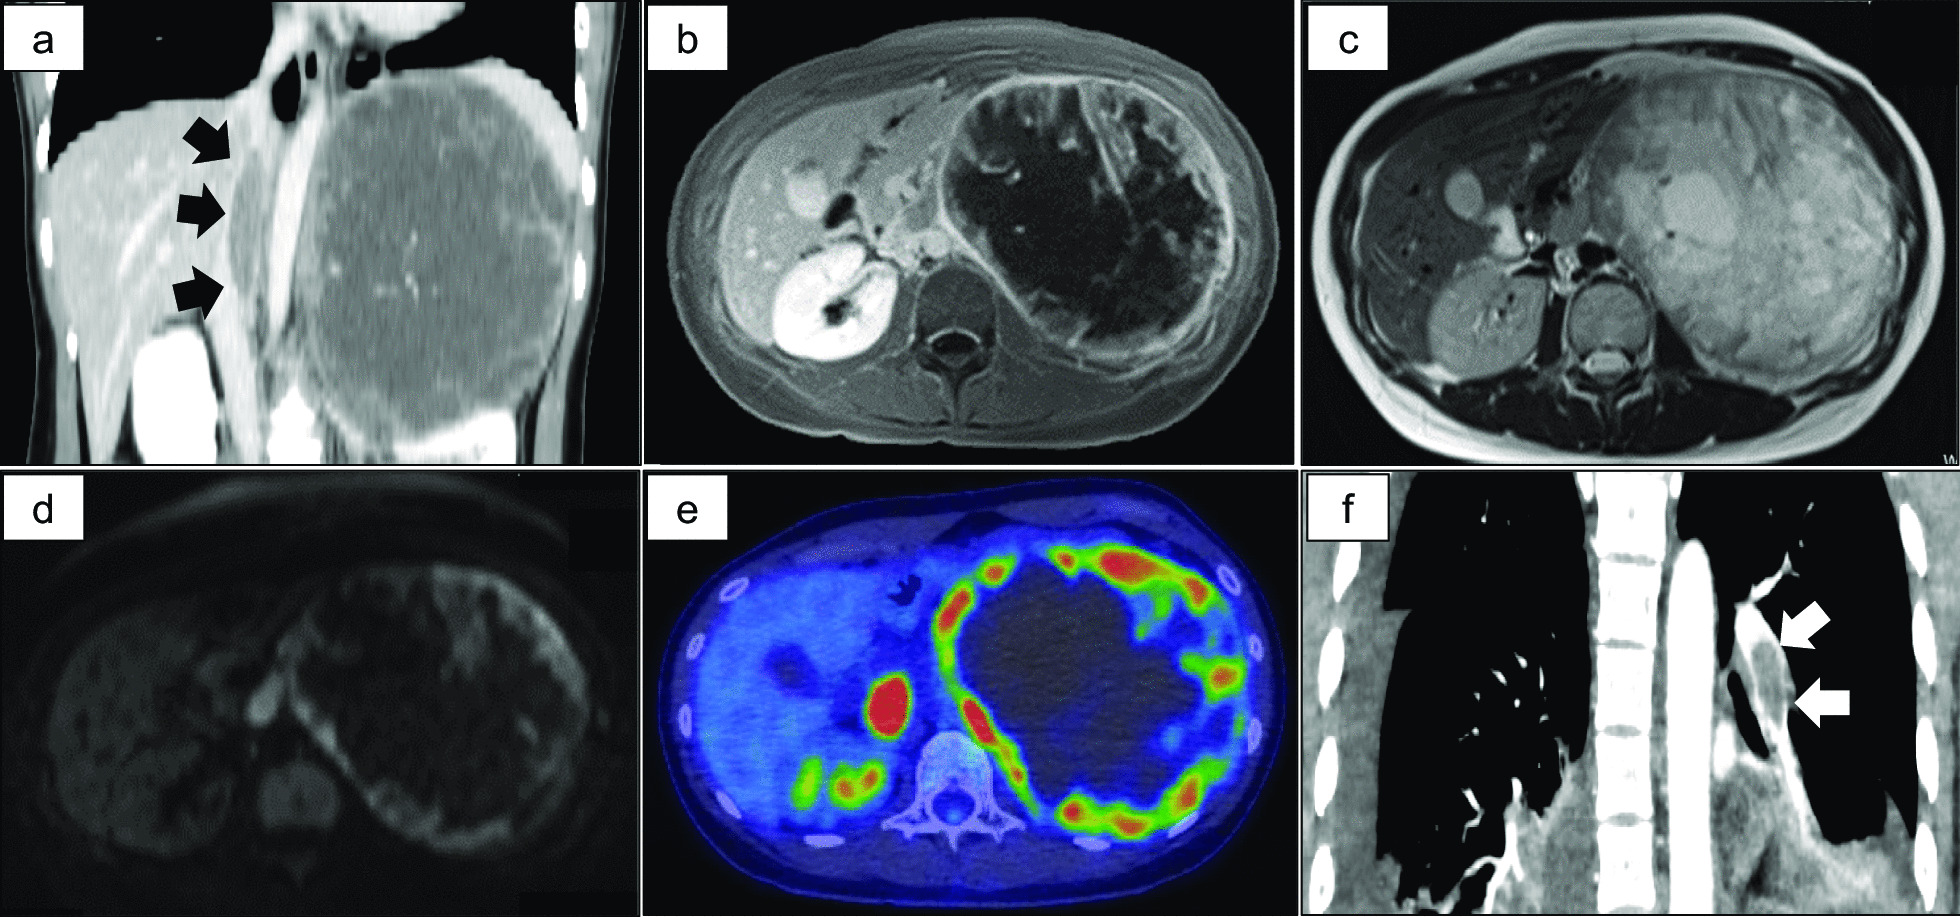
Preoperative CT, MRI, and FDG-PET. a Contrast-enhanced CT reveals irregular contrast in a left retroperitoneal tumor 19 cm in diameter, showing tumor thrombus in the left renal vein, adrenal vein, and vena cava. Black arrows indicate tumor thrombus within the inferior vena cava. b–d MRI reveals an encapsulated multilocular tumor with partially solid component, showing hypointensity on T1-weighted imaging, irregular hyperintensity with a septum on T2-weighted imaging, and strong signals on DWI. e On ^18^F-FDG-PET–CT, maximum standardized uptake value is 12.2 and no distant metastases are evident. f Contrast-enhanced thoracic CT on day 4 after surgery reveals tumor embolization in the left pulmonary artery (white arrow)
